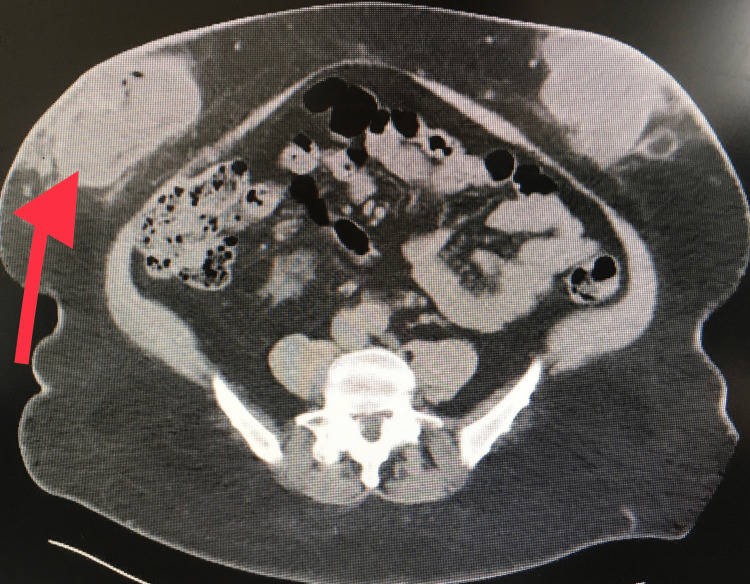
CT of the abdomen Rim-enhancing right lower quadrant mass concerning for abscess or hematoma (red arrow).
